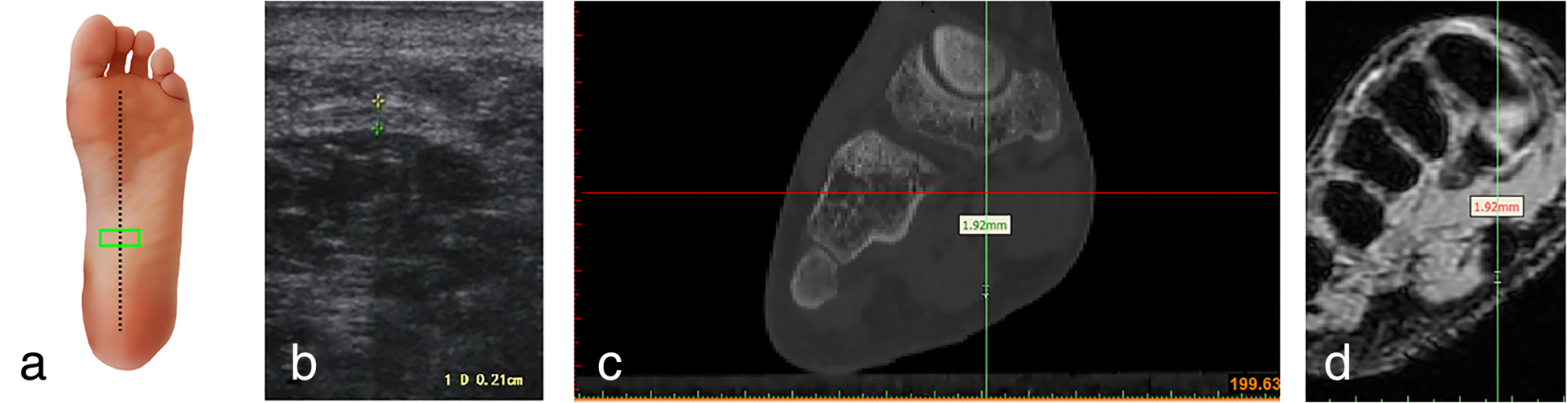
Ultrasound, CT and MRI measurements of the arch of the plantar fascia (transverse section) a Ultrasonic probe is placed (green panel). b Ultrasonic measurement. c Measuring the thickness of the plantar fascia reconstruction CT images. d Measuring the thickness of the plantar fascia reconstruction MRI images
